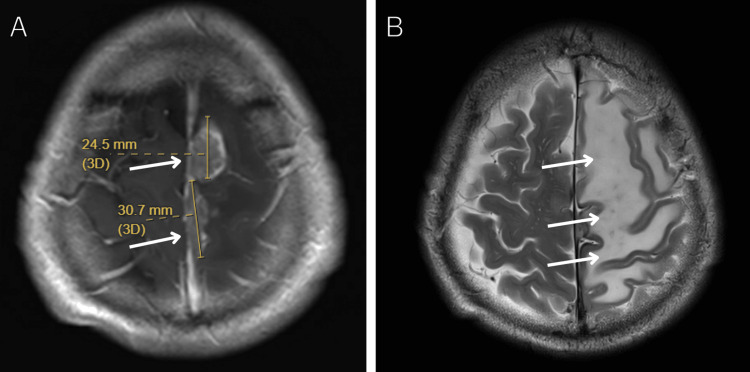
Disease progression on osimertinib monotherapy. (A) Axial T1-weighted contrast-enhanced MRI brain image showing progressive parasagittal lesion after whole-brain radiotherapy while on continued osimertinib monotherapy. Measurements indicate tumor dimensions of 24.5 mm and 30.7 mm. (B) Axial T2-weighted MRI brain image showing significant perilesional edema, demonstrating continued disease progression despite osimertinib treatment.
